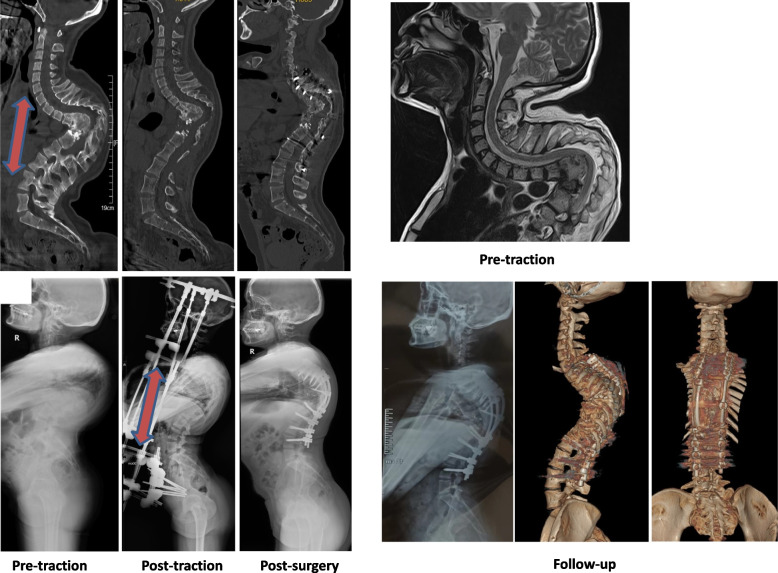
A 31-year-old male presented with a 17-year history of tuberculous angular kyphosis. The patient complained of back pain, difficulty breathing, and weakness of both lower extremities (ASIA D). Preoperative CT demonstrated extensive fusion of the vertebral bodies from T6-T12, and MRI showed the spinal cord had been drawn into the angular kyphosis region. After 12 weeks of HPT, the kyphosis angle decreased from a pre-traction value of 145° to a post-traction value of 87°. CT showed an abruption at the distal end of the kyphosis (between L1 and T12); however, neurological symptoms did not become worse. Posterior decompression, pedicle screw fixation (T1-L4), PSO, and autologous bone grafting were performed via a posterior approach to achieve final correction. Postoperatively, the kyphosis angle was 75°. By 3 months after the operation, the patients breathing difficulty and back pain were completely relieved. The numbness in the lower extremities gradually diminished and was resolved by 6 months after the operation (ASIA E). The red arrow showed the orientation of the traction force
